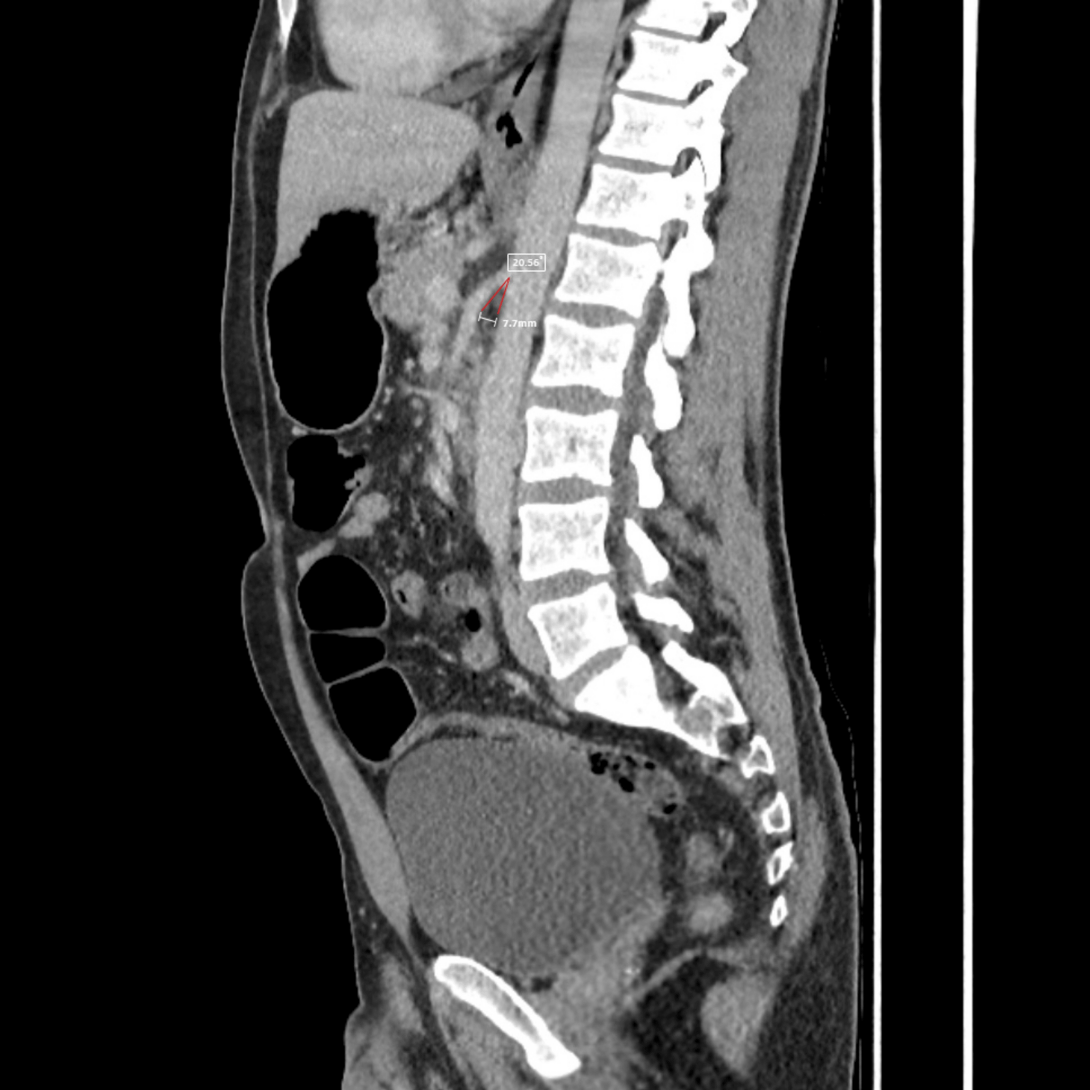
CT of the abdomen and pelvis with contrast, sagittal slice, showing a decreased aorto-mesenteric angle of 20 degrees (measured) and decreased aorto-mesenteric distance of 7.7 mm, suggestive of superior mesenteric artery syndrome.
